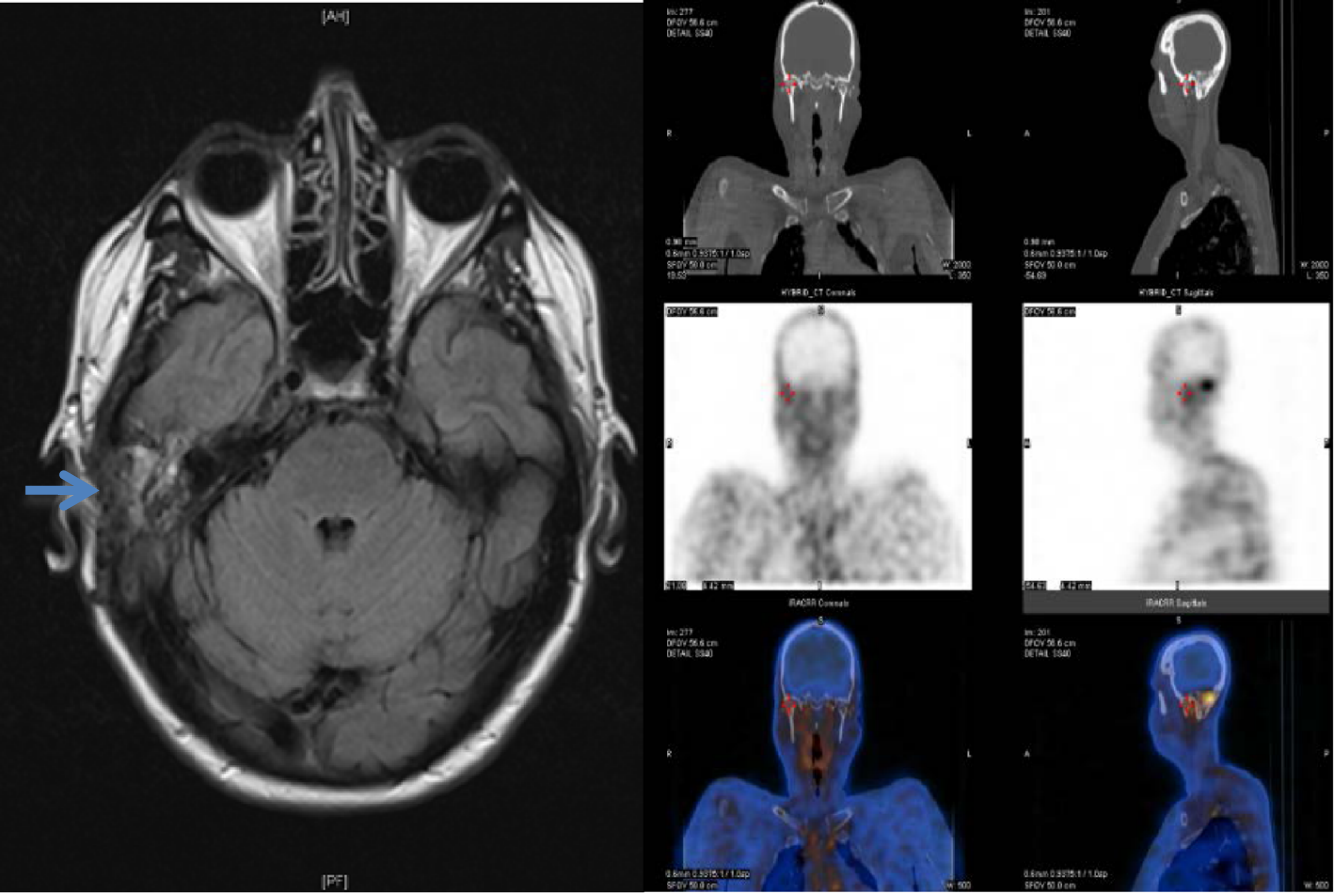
MRI scan - T2 weighted image showing soft tissue oedema in the region of the right mastoid (left-see arrow) and technetium 99 m scan showing increased uptake in the same area (right).
